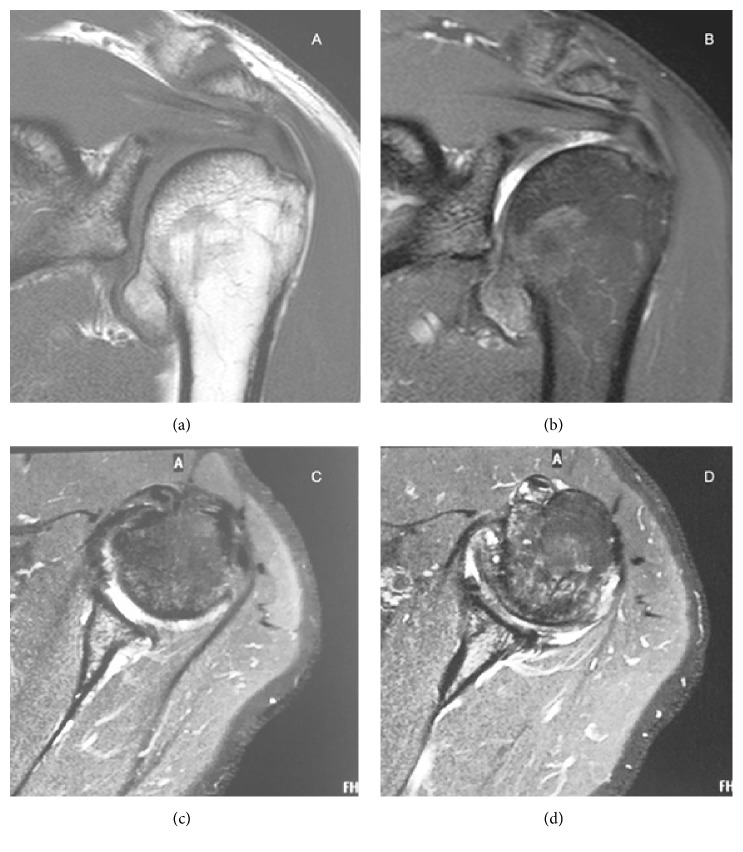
MR images of the left shoulder revealed the inferomedial mass projecting toward the inferior capsular recess, with sharpening of the articular cartilage of the humeral head, but no signs of OA. Cartilaginous components of the tumor showed intermediate T1-weigthed signal and hyperintense proton density- (PD-) weighted signal. In contrast, bone marrow of the tumor showed hyperintense T1-weighted signal and intermediate-to-low PD-weighted signal. (a) T1-weigthed coronal image, (b) PD-weighted coronal image, and (c) and (d) T2-weigthed axial images.
